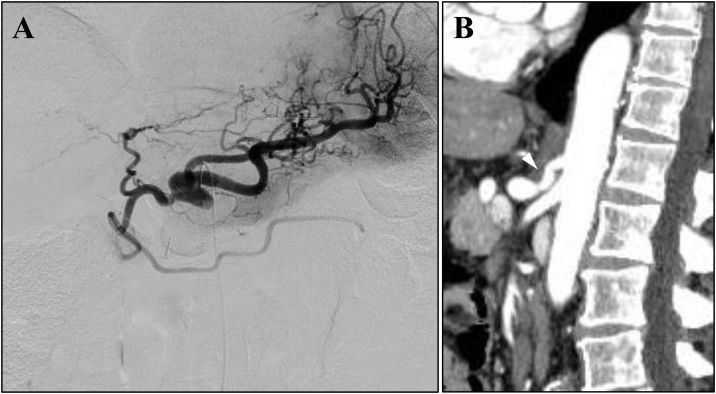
The findings of intraoperative angiography and postoperative follow-up CT: (A) The CA angiography after the MAL release without ganglionectomy of the celiac plexus showed the antegrade blood flow to the proper hepatic artery clearly. (B) The follow-up CT performed 12 months after the surgery revealed no residual CA stenosis (white arrowhead).
